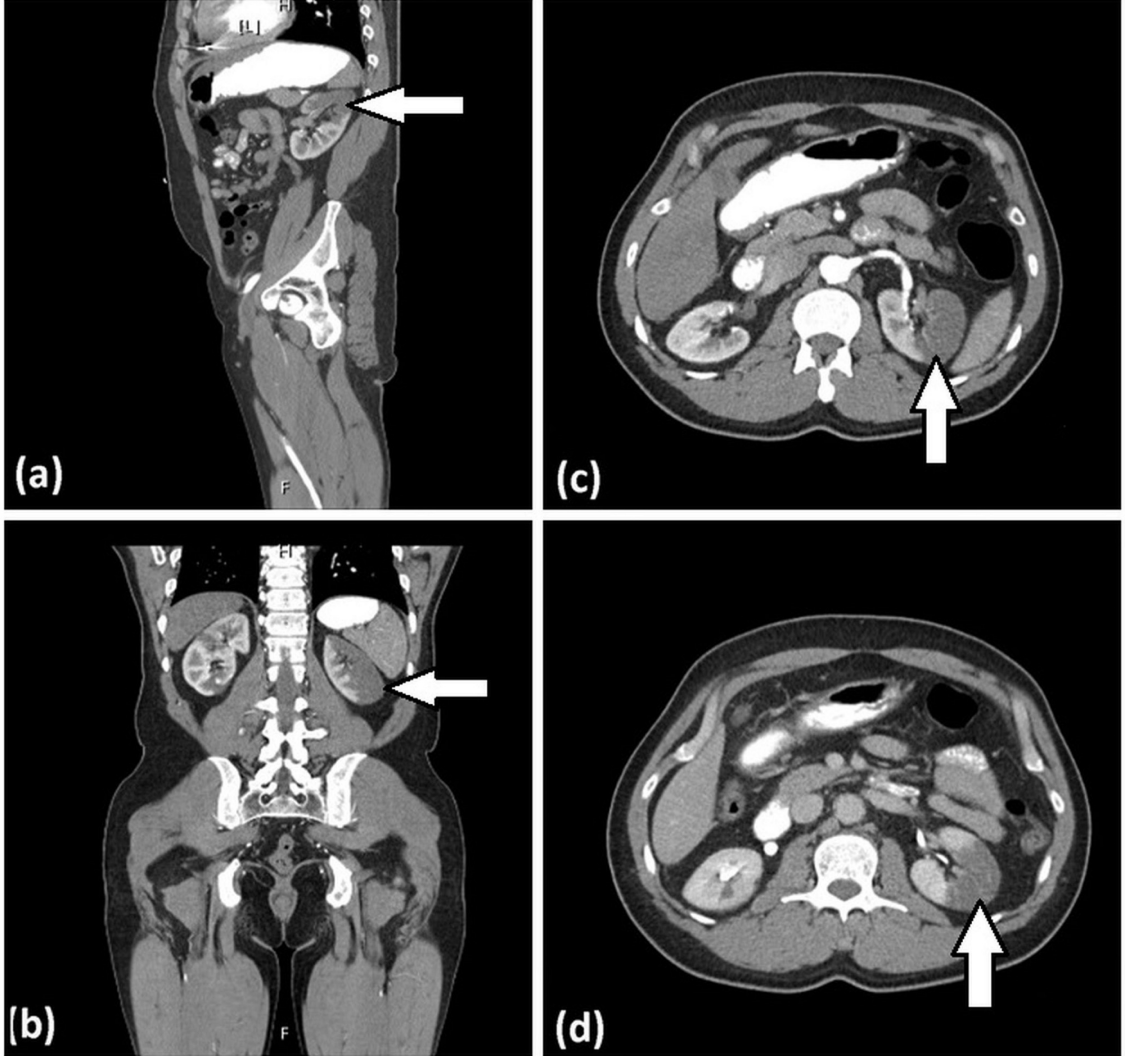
Computed tomography (CT) of the abdomen and pelvis with contrast Figure 1 shows a bilateral renal infarction. The arrows depict filling defects in the (a) sagittal, (b) coronal, and (c, d) axial images of the CT scan of the abdomen and pelvis with contrast.
